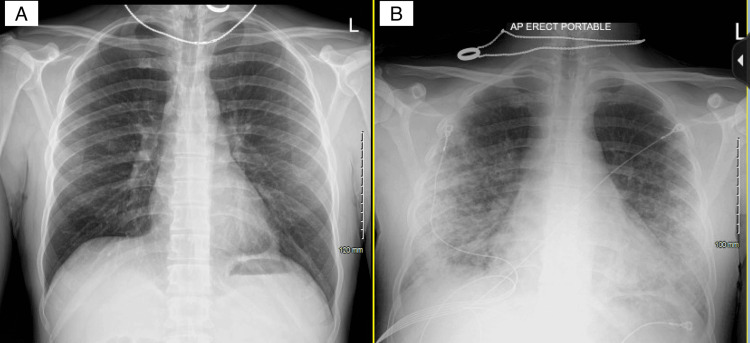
Comparison of the chest X-rays taken on the day of admission and day three of hospitalization A: The initial chest X-ray was unremarkable; B: Chest X-ray on day three of hospitalization shows bilateral airspace opacities
